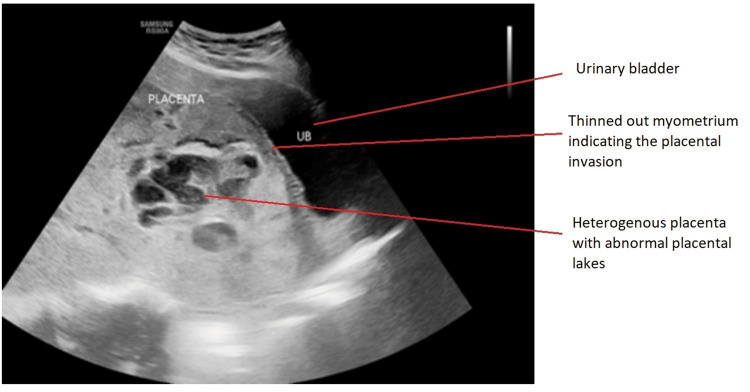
USG scan of the placenta USG: ultrasonography
